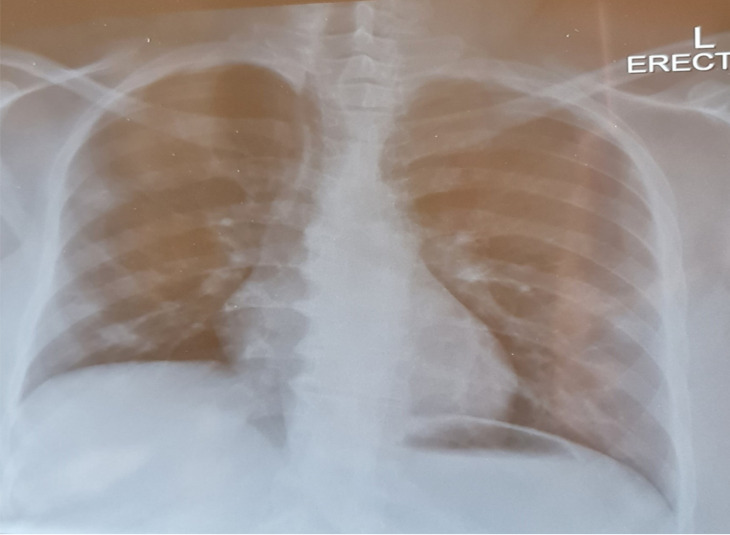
Chest x-ray on day of admission (Source: Created by Author)
